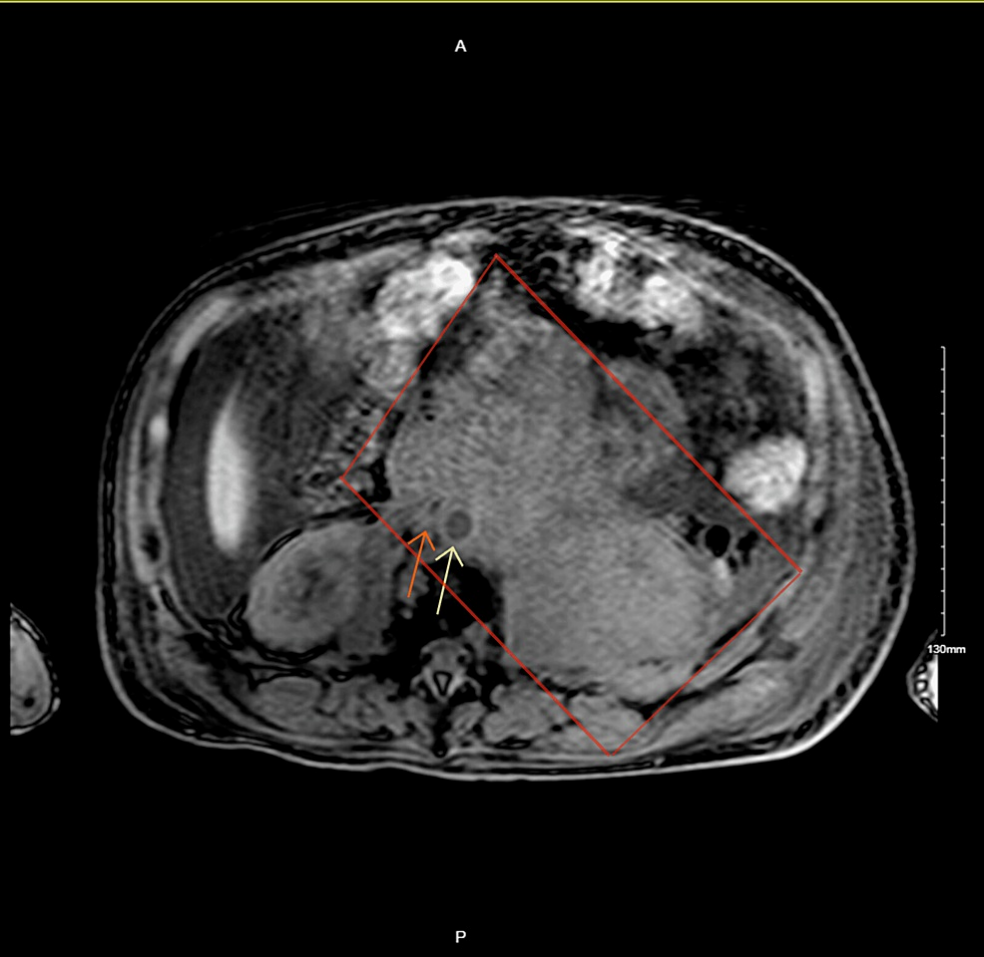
MRI of the abdomen without contrast showing a large infiltrative left retroperitoneal mass. It surrounds the aorta (yellow arrow), and there is a narrowing of the lumen of the inferior vena cava (orange arrow), indicating a mass effect. MRI: magnetic resonance imaging
